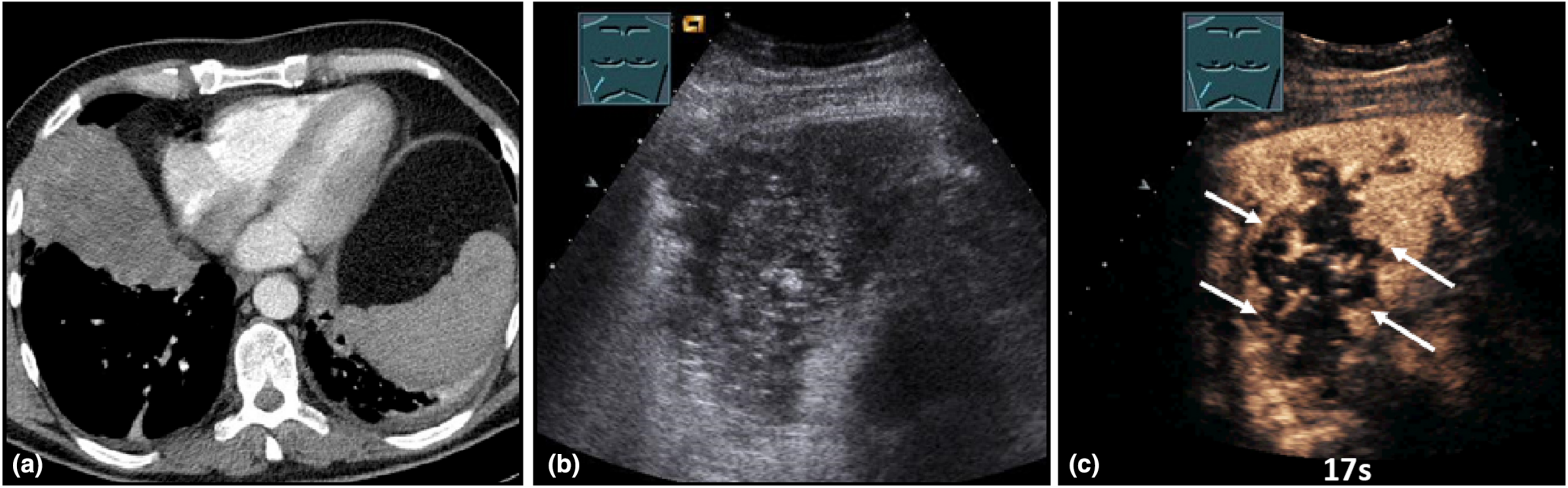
A 55‐year‐old male patient presented to the emergency department with respiratory distress due to aspiration of fire‐eater oil. Visualisation of a right‐sided, homogeneous, middle‐lobe consolidation on computed tomography (courtesy of Prof. Dr. Andreas H. Mahnken, Department of Radiology, University Hospital Marburg) (a); B‐mode ultrasound (b) shows a hypoechoic, irregularly bordered, inhomogeneous consolidation; contrast‐enhanced ultrasound (c) shows a pulmonary arterial perfusion after 17 seconds with a central non‐enhanced area (arrows), suggesting the presence of aspiration pneumonia with a complete regression after antibiotic therapy.
